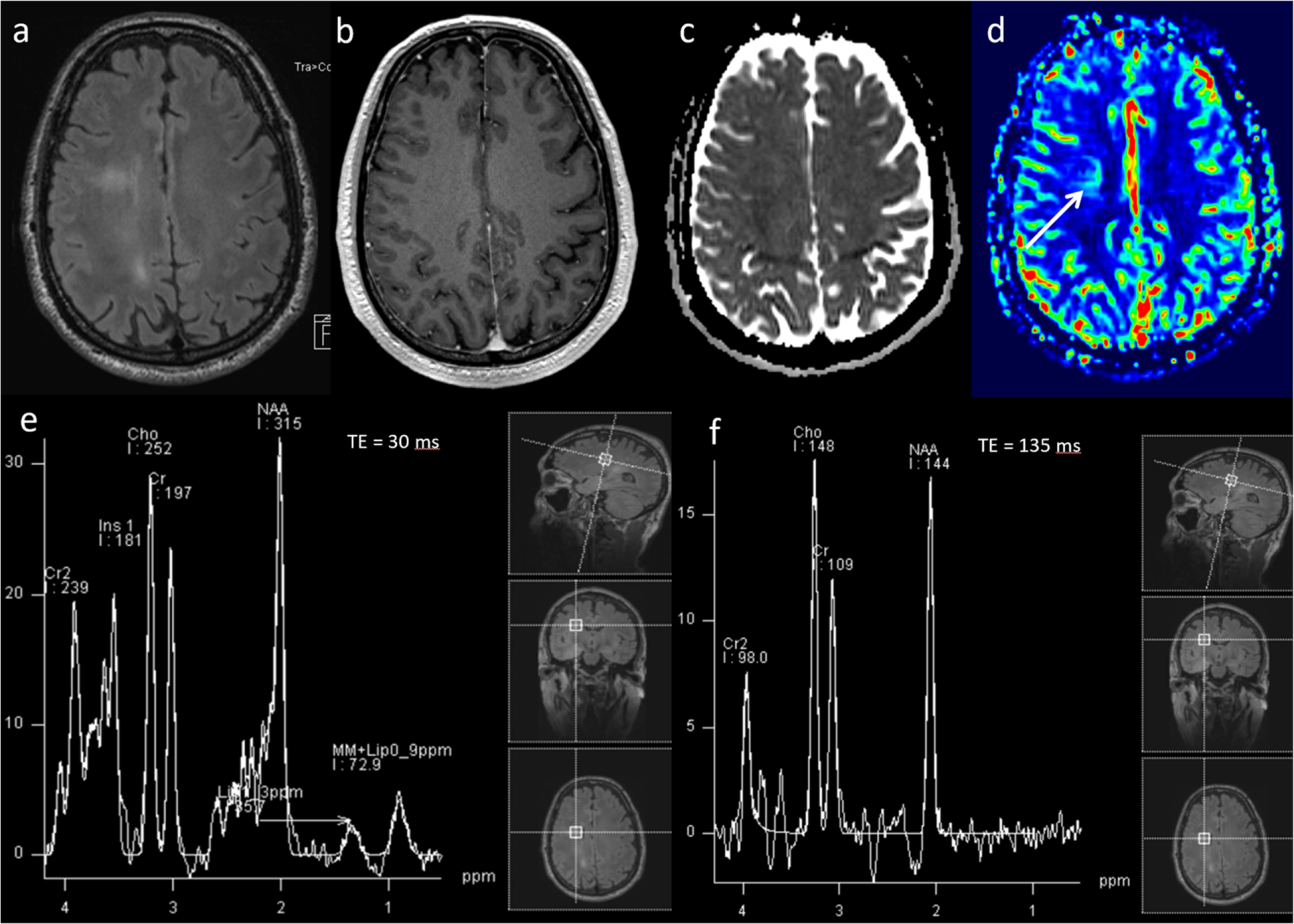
Gliomatosis cerebri. Conventional MRI: a, b Axial FLAIR and post-contrast T1W sequences, showing diffuse infiltrative non-enhancing deep white matter lesion. Multiparametric MRI: c ADC map demonstrates no areas of low ADC. d However, PWI shows a focal area of slightly raised perfusion in the right frontal centrum semiovale (arrow) compared to normal-appearing white matter. e, f MRS shows high mI/Cr ratio, slightly raised Cho/Cr ratio (1.2) and slightly low NAA/Cr ratio. Focal raised perfusion and choline area was chosen for the optimal site of biopsy
